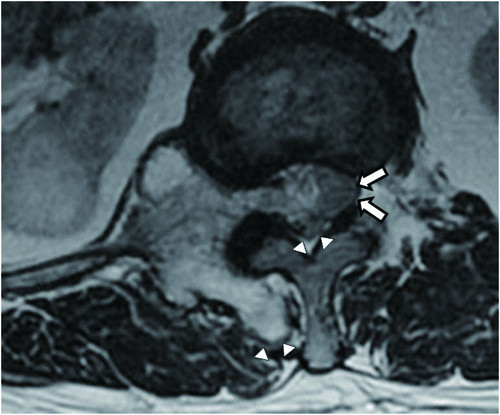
Axial T2-weighted MR image. The image shows an intraspinal osseous mass causing compressive myelopathy (arrows). Note 2 areas of the thin cartilaginous cap (arrowheads) on both the posterior and the intraspinal aspects of the mass.
